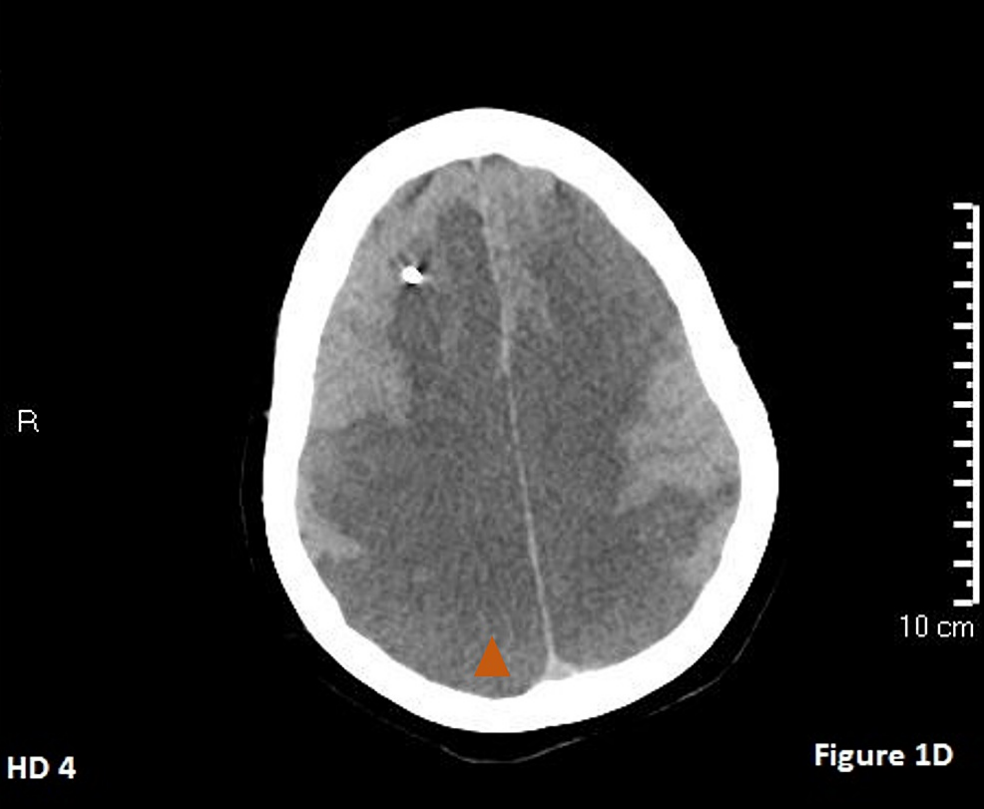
Hospital day 4 CT of the head. The orange triangle points to the hypodense region showing the progression of bilateral cerebral edema worse than the previous day.
